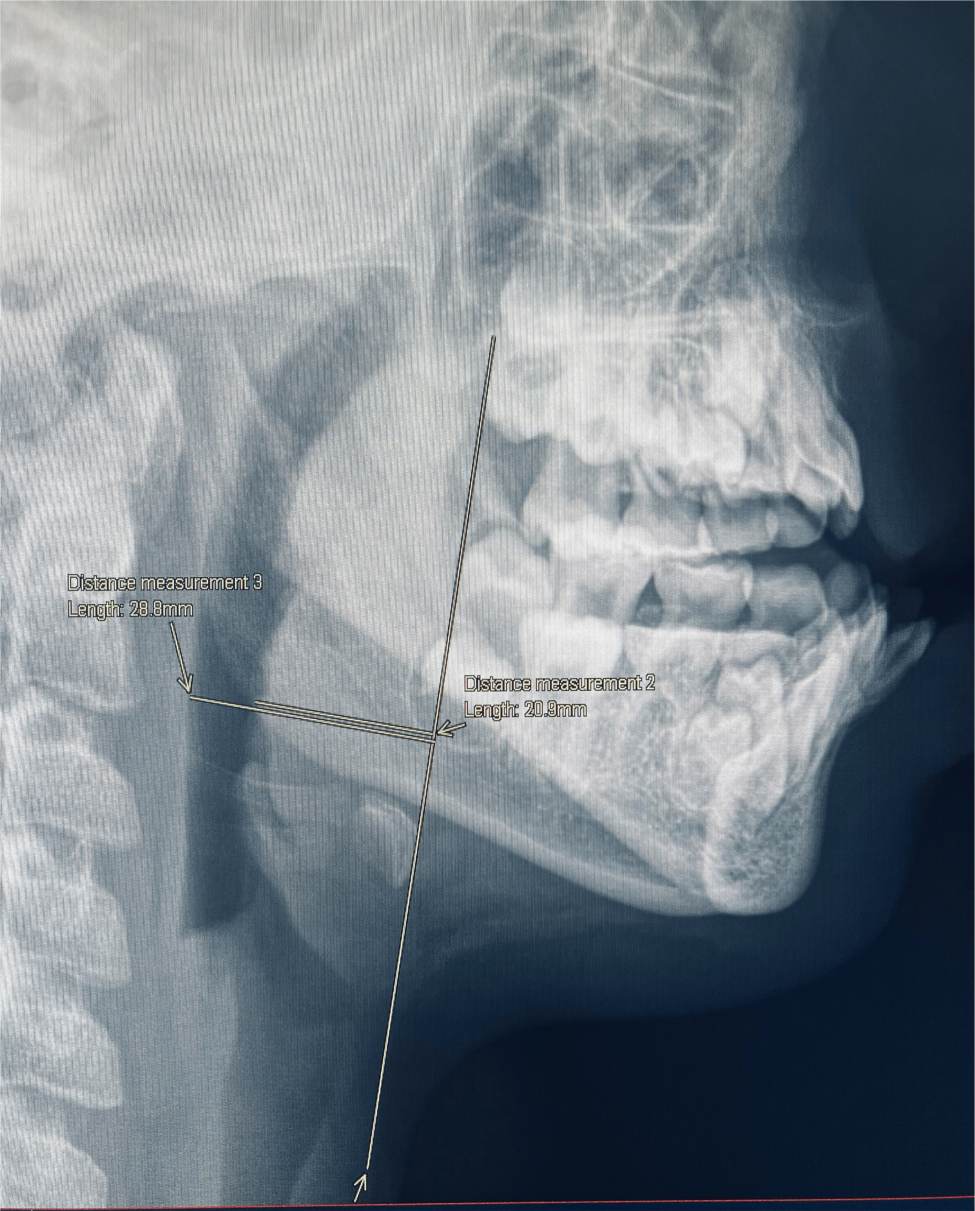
X-ray demonstrating the objective measurement of the BOT A vertical line (baseline) is drawn from the posterior bony edge of the hard palate tangentially over the anterior surface of the hyoid bone. The hypopharyngeal airway (A): line extending from the posterior pharyngeal wall to the baseline. The thickness of BOT (B): line from maximum convexity to baseline. BOT: base of tongue
